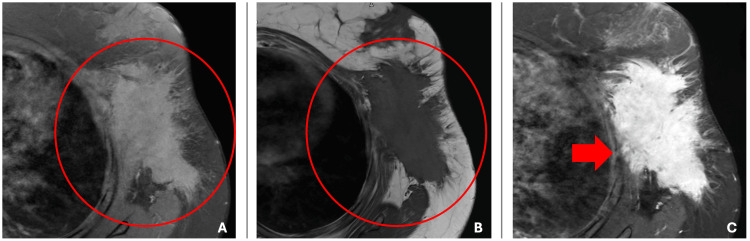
Axial contrast enhanced MRI scans of the left breast on 3T (A) Axial T1-weighted imaging showed the mass to be hypointense (repetition time scan/echo time msec, 636/10, section thickness 6 mm). Axial T1-weighted turbo spin echo (B) without fat saturation (repetition time scan/echo time msec, 636/10, section thickness 6 mm) and (C) with fat saturation (repetition time scan/echo time msec, 640/10, section thickness 6 mm) showed the mass to be within the breast with the involvement of the adjacent pectoralis, anterior serratus, and latissimus dorsi musculature, and no distinguishable intervening fat plane showing fascial involvement (red arrow).
